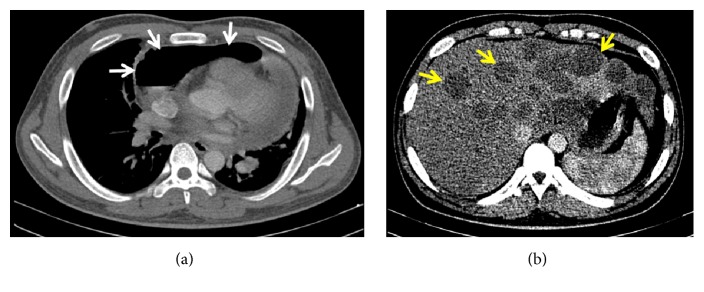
CT scan images. (a) Cross-sectional cut of the heart and pericardium revealed an air-fluid level within the pericardial sac (white arrows). (b) Cross-sectional cut of the liver showed multiple hypodense nonenhancing foci (yellow arrows).
